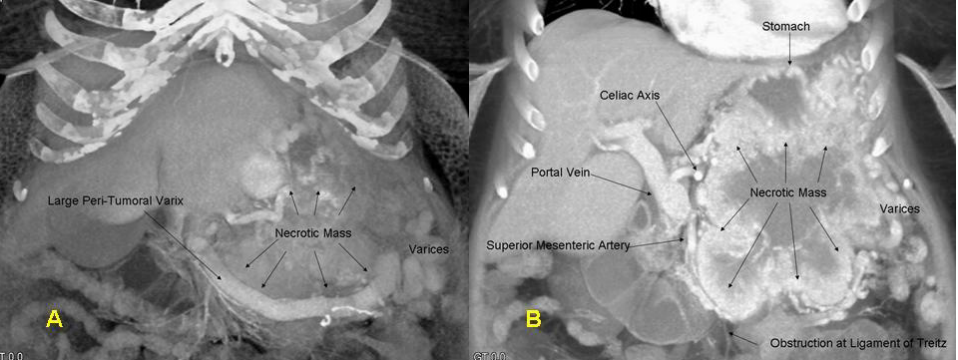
(A) 3-D CT coronal reconstruction showing the pancreatic VIPoma, a large peri-tumoral varix, and gastric varices. (B) 3-D CT coronal reconstruction depicting relation of pancreatic VIPoma to adjacent vascular structures and stomach. Note presence of varices as well as invasion of tumor into the fourth portion of the duodenum.
